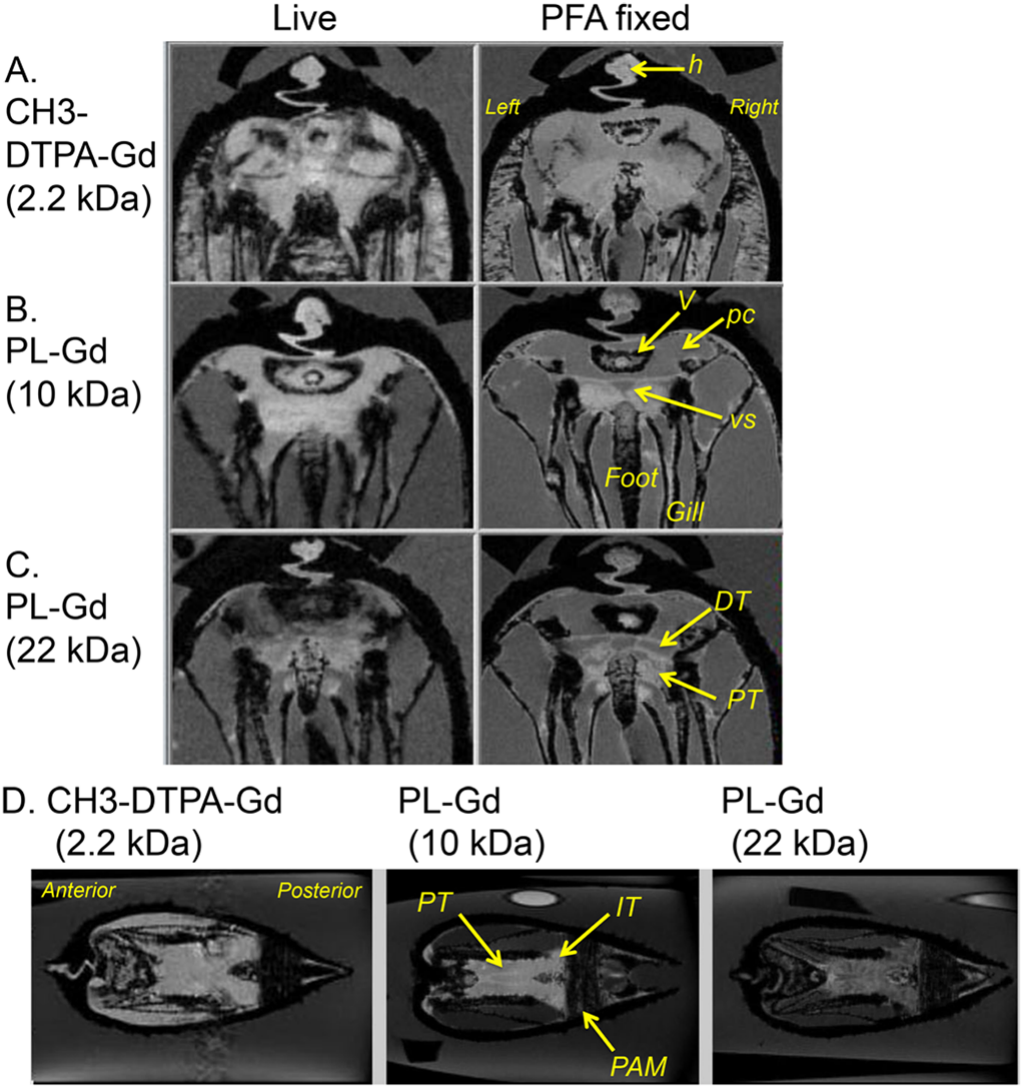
Distribution of injected MR tracers in *N. douglasiae* imaged by *T*_1w_-MRI. (A–C) The left column shows a transverse image at 1 mm anterior from the atrioventricular valve after the injection of (A) CH3-DTPA, (B) 10 kDa PL-Gd, and (C) 22 kDa PL-Gd. Regions of higher signal intensity compared with the water indicate the presence of the MR tracers. The right column shows a corresponding high resolution *T_1w_-*MRI of the PFA fixed mussel. (D) Coronal images at the level of the PT after injection of the MR tracers. DT, the distal tubule; h, hinge; IT, the intermediate tubule; PAM, posterior adductor muscle; pc, pericardial cavity; PT, the proximal tubule; V, ventricle of the heart; vs, the visceral sinus.
